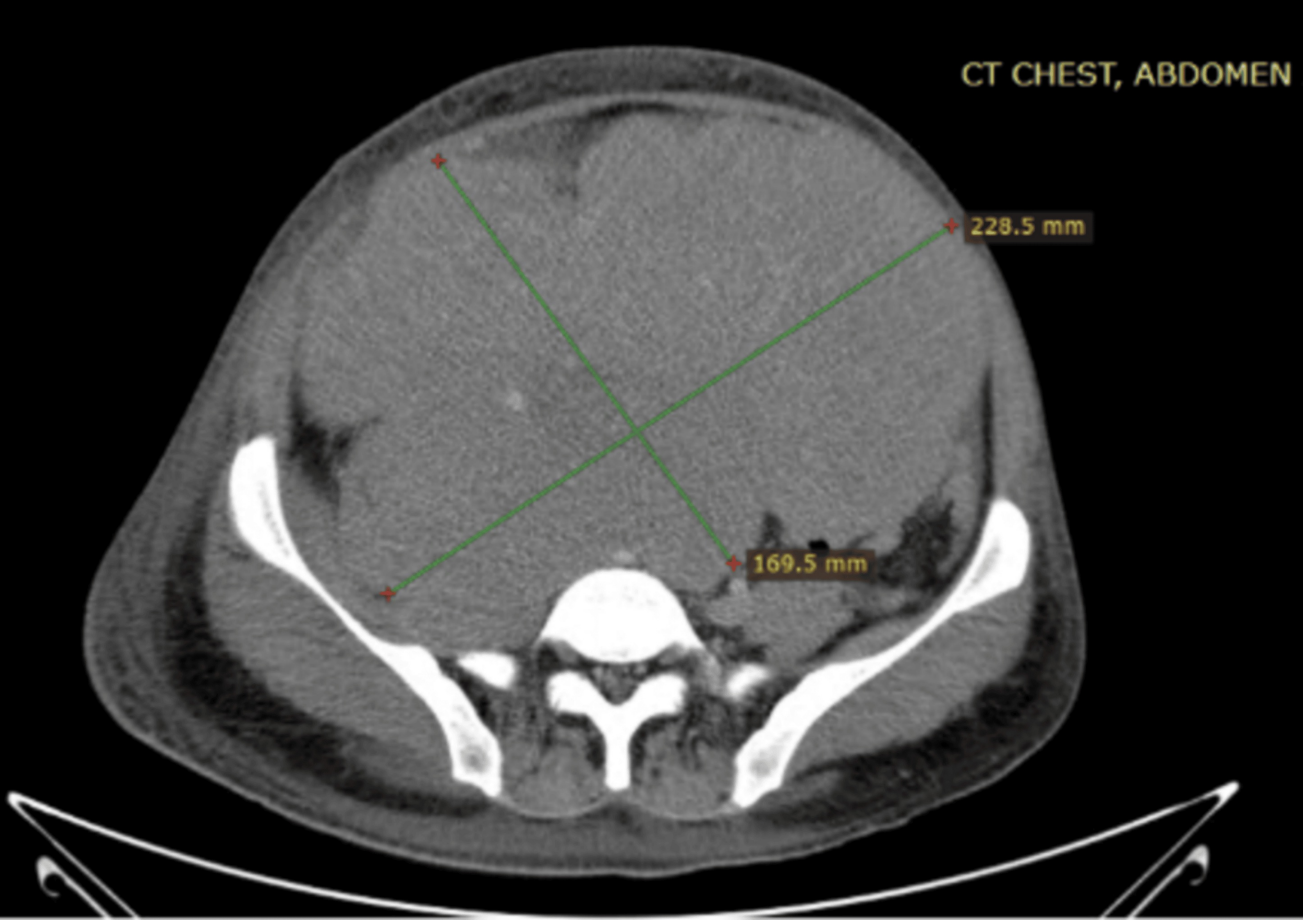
Axial image of a CT scan showing around 23×17 cm mass in the pelvis CT: computed tomography
